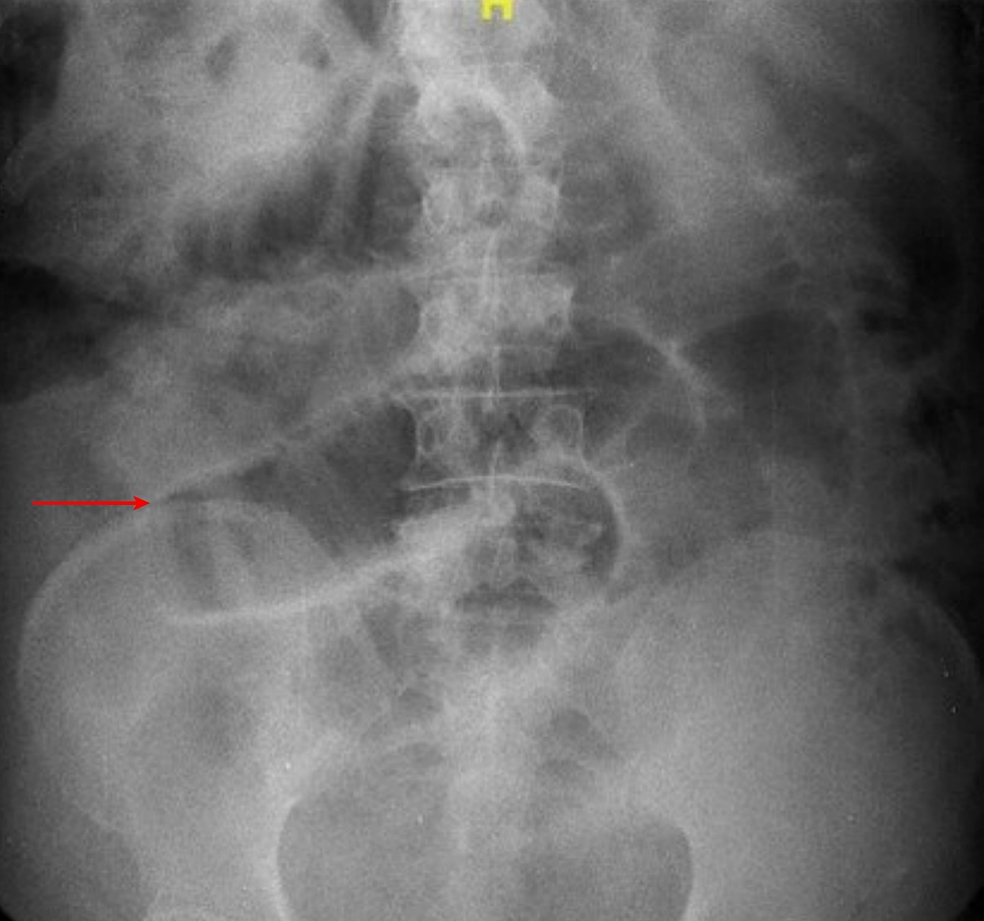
X-ray of the abdomen showing dilated jejunal loops (red arrow).
